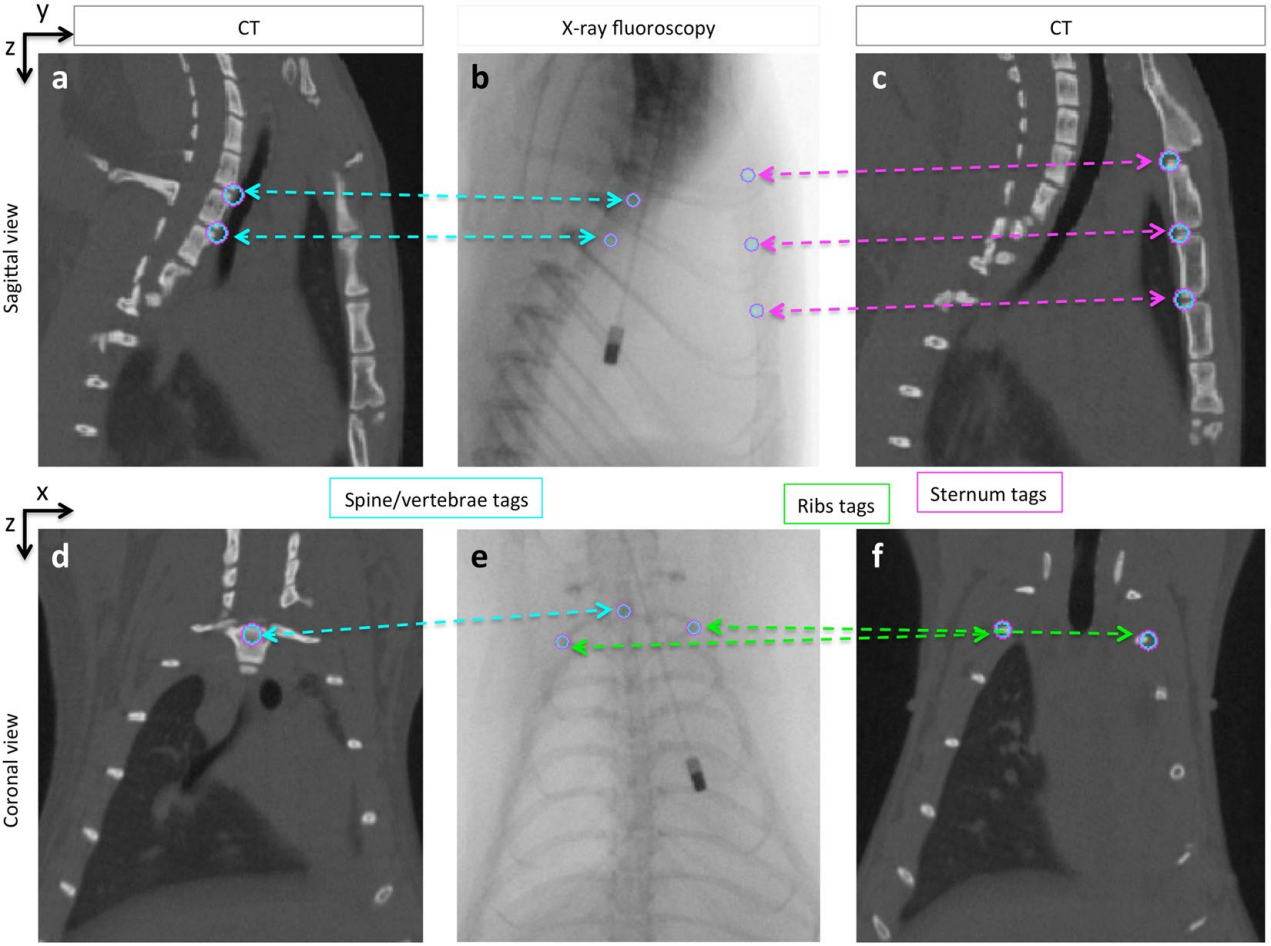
X-ray fluoroscopy to CT registration with point-matching method. Sagittal view (a,b and c) and coronal view (d,e and f) showing matching tags (circles) placed on anatomical landmarks appearing on both CT (a,c,d and f) and fluoroscopy (b and e). Tags are placed on visible structures such as the spine or vertebrae (cyan arrows), the ribs (green arrows) or the sternum (magenta arrows). The sagittal view provides the y and z coordinates and the coronal view provides the x and z coordinates.
